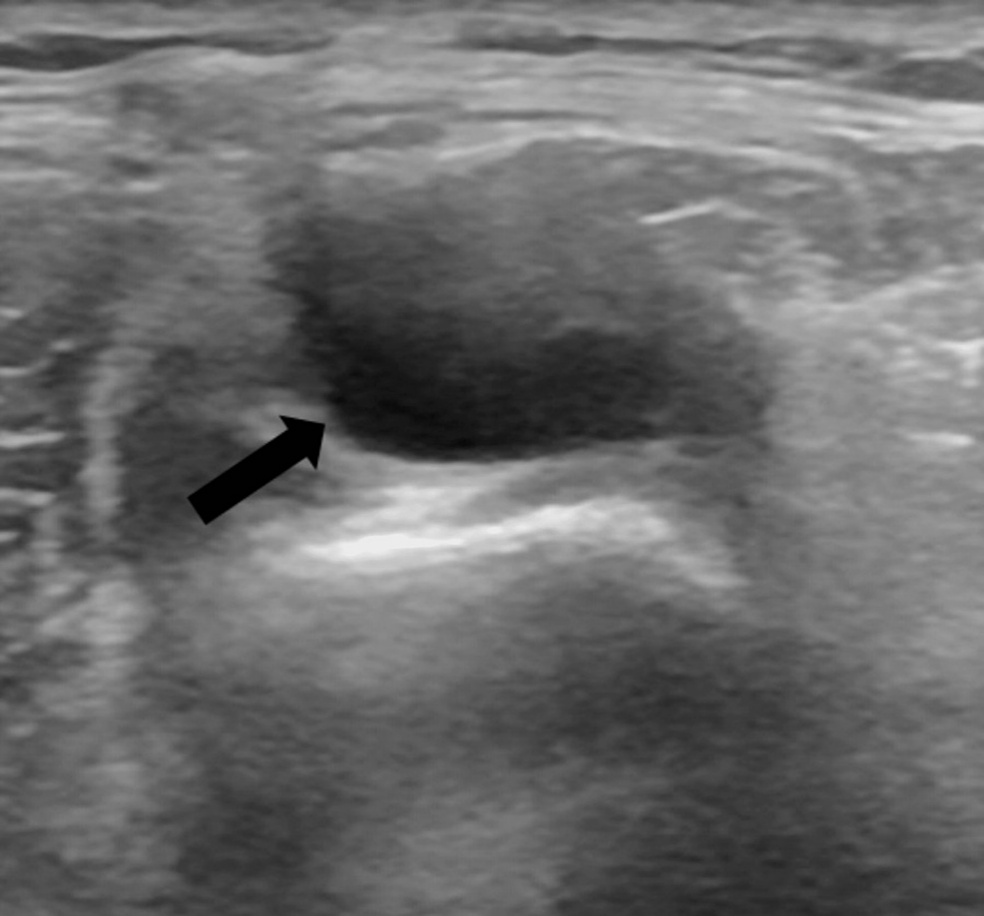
Initial ultrasound of the right lower extremity demonstrated the solid and cystic soft tissue mass (black arrow) originally found near the level of the fibular tunnel
